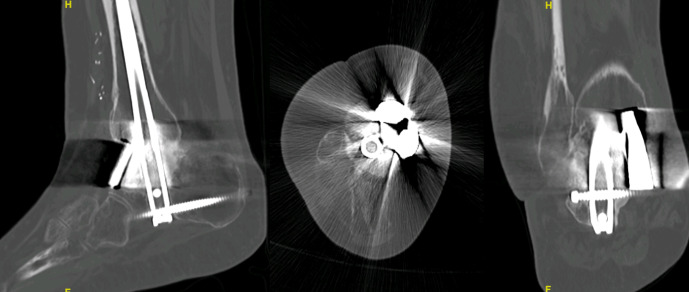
In spite of the image distortion due to radiolucent osteosynthetic material, signs of consolidation and the absence of indirect signs of pseudoarthrosis can be noted in the 1-year control CT scan of one of the patients (Case 2). This image, along with a painless weight-bearing ambulation, is enough to consider a consolidation of the defect.
